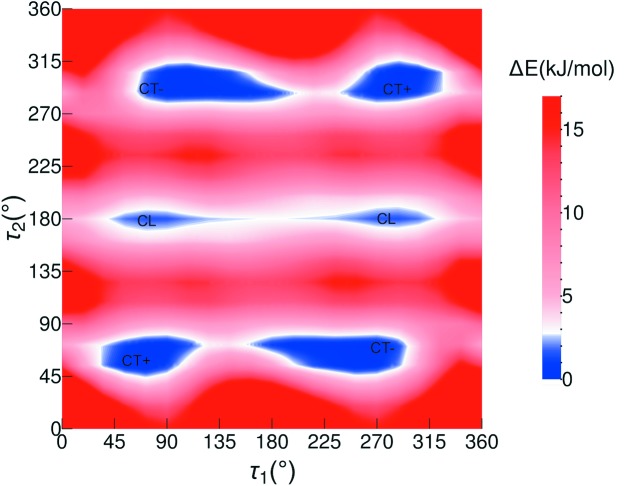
Conformational PES (B97d/6-311G) of 2PrBzIm as a function of torsion angles τ_1_ and τ_2_. Positions of conformers CL, CT+ and CT− are labelled.
